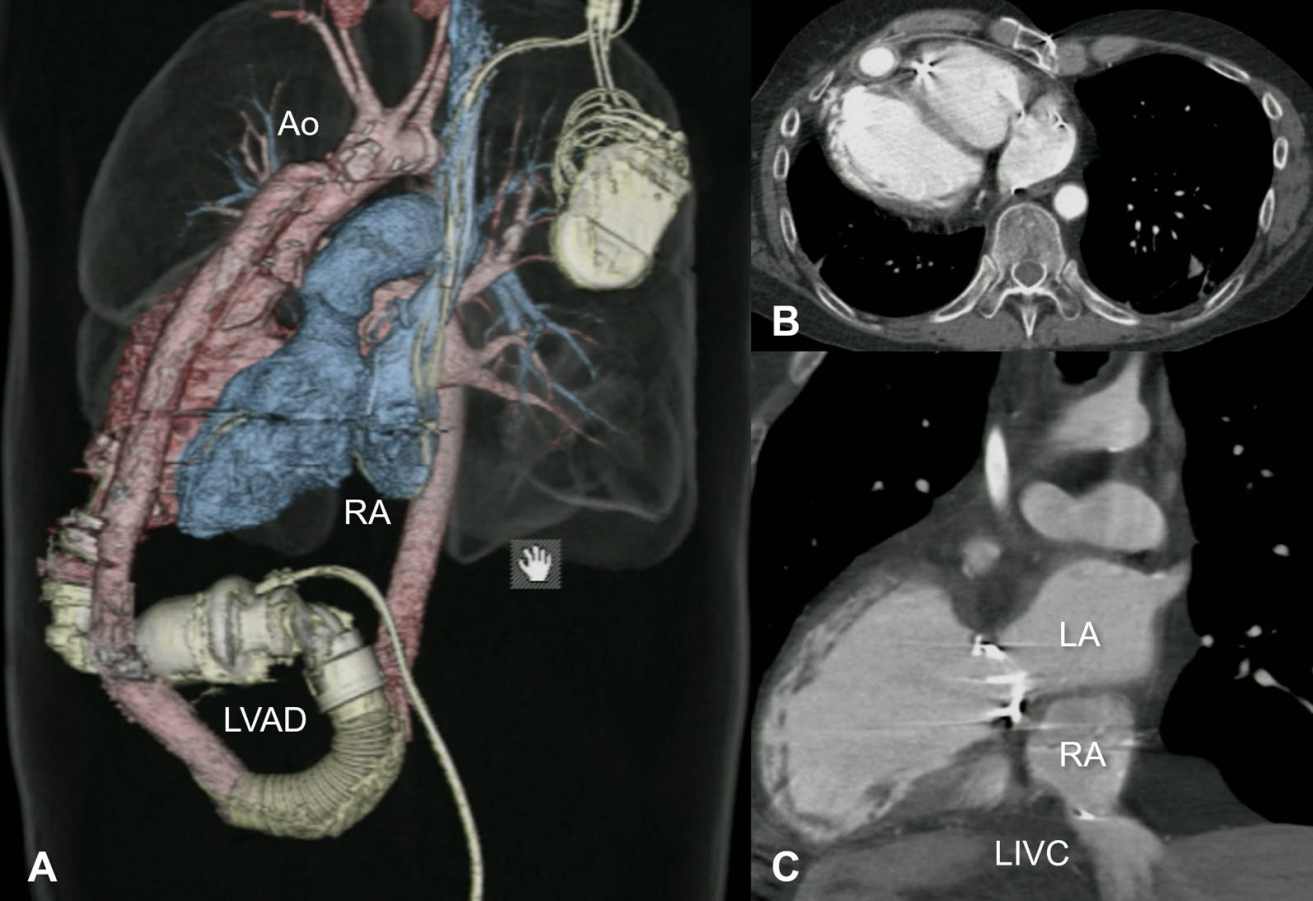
Preoperative computed tomography images: a 3D image showed HeartMate II implanted in a reversed fashion (A); an axial image demonstrated a chest deformity (B); a coronal image presented the relationship between LA, RA, and LIVC (C). *Ao* aorta, *LA* anatomical left atrium, *LIVC* left inferior vena cava, *LVAD* left ventricular assist device, *RA* anatomical right atrium
